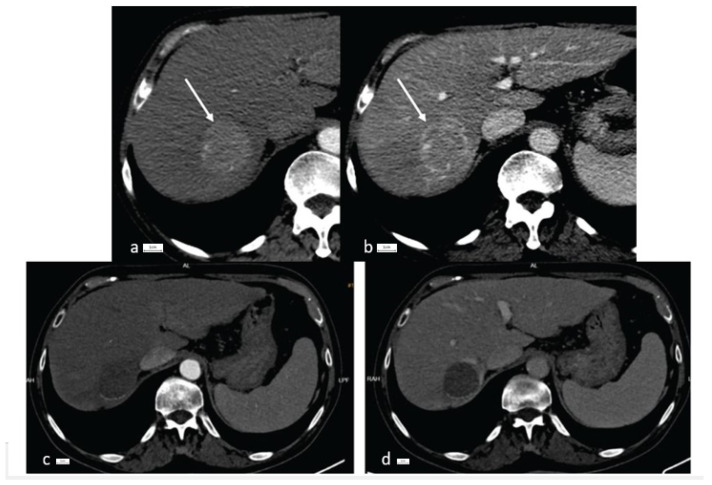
CT imaging of HCC in an 84-year-old patient before and after interventional therapy. Initial contrast-enhanced CT showing a 45/50 mm tumor (arrow) in the 7th segment, with arterial hyperenhancement (a) and portal wash-out (b). CT imaging after TACE and RFA showing no hyperenhancement in the arterial phase (c) and no wash-out in the portal phase (d).
